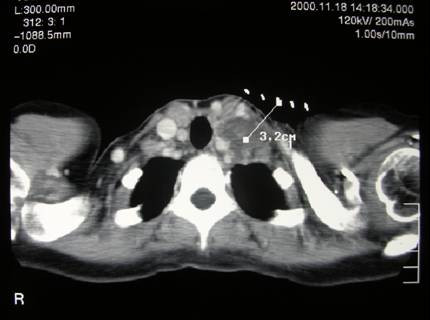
Computed tomography showing a low density lesion with irregular and thick margins in lateral neck region.
